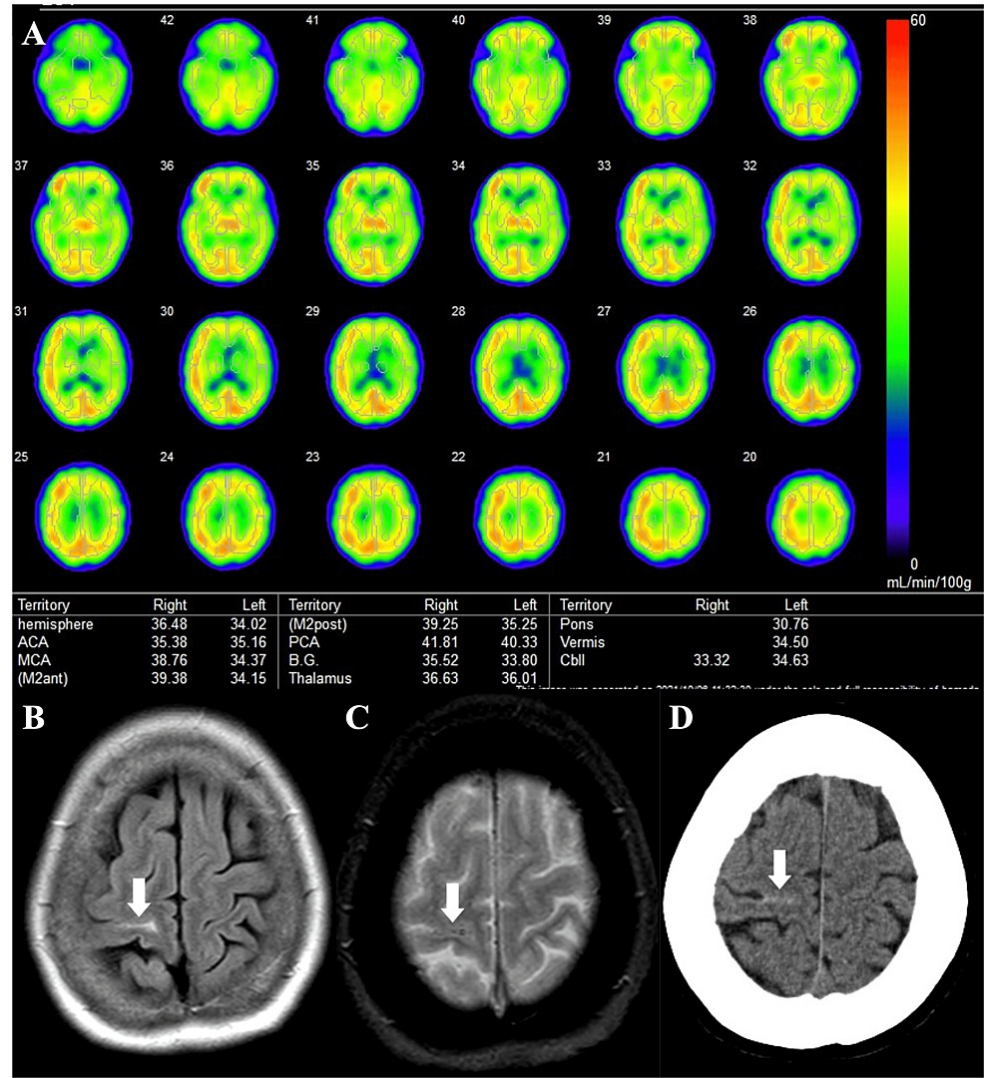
Radiological imaging after carotid artery stenting Quantitative cerebral blood flow measurements using N-isopropyl-(iodine 123) p-iodoamphetamine and single photon emission computed tomography (SPECT) on two days after carotid artery stenting (A) showed no obvious increase in cerebral perfusion on the operative side. Fluid attenuated inversion recovery (FLAIR) imaging (B) and T2*-weighted image (C) on magnetic resonance imaging (MRI) performed seven days after carotid artery stenting and computed tomography (CT) imaging eight days after stenting (D) showed small amount of subarachnoid hemorrhage in her right central sulcus (arrow).
